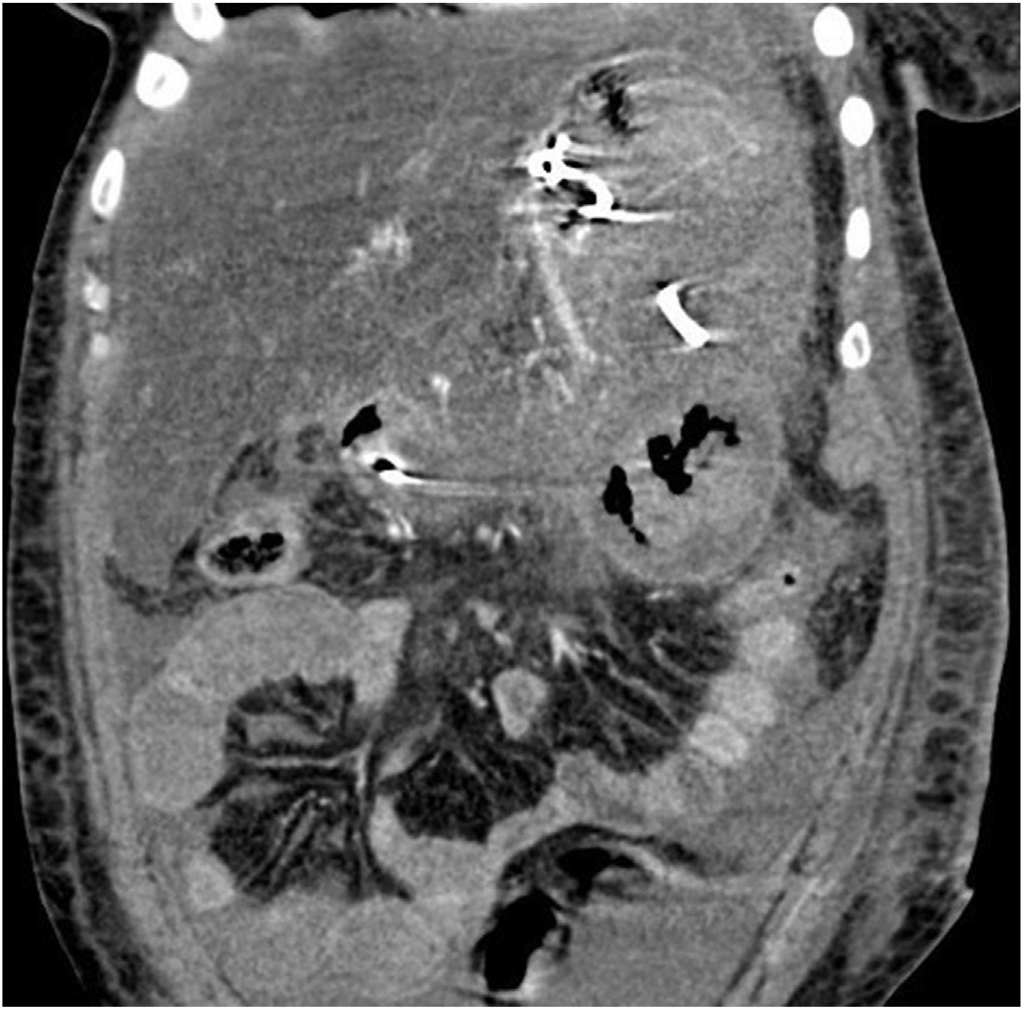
Computed tomography angiography abdomen and pelvis showing blood products in same walled-off collection.
